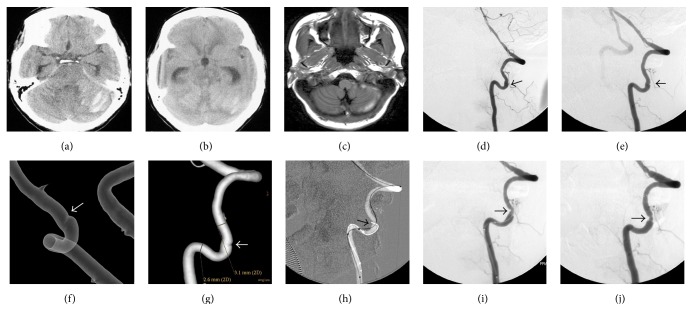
CT (a) showed lamellar infarct in the pons and worm of cerebellum and SAH. CT (b) showed obstructive hydrocephalus. MRI (c) showed SAH gradually decreased after ventriculoperitoneal shunt. Lateral and oblique DSA ((d) and (e)) of left VA showed the dissection in the segment of V2. Transparency and volume rendering ((f) and (g)) showed the intimal flap. It is the major appearance. During the procedure, the micro-guide wire was difficult to pass through the true lumen until the microcatheter was used (h and i). Lateral DSA showed slight remnant stenosis after stenting (j). CT: computed tomography; MRI: magnetic resonance image; DSA: digital subtraction angiography; VA: vertebral artery.
